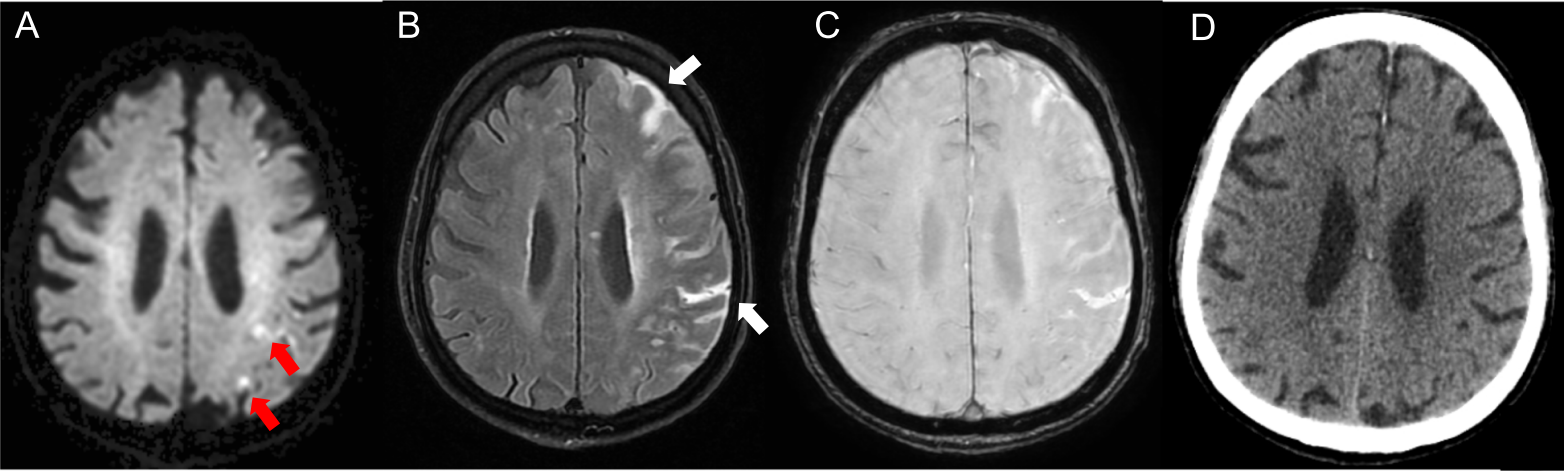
Day 2: Hyperintense Acute Reperfusion Marker Sign Associated with Acute Ischemic Lesions, Without Evidence of Subarachnoid Hemorrhage. A DWI sequence shows recent ischemic lesions (red arrows). B Non-enhanced FLAIR MRI sequence shows left hemispheric sub-arachnoid hyperintensity (white arrows). C SWAN MRI sequence shows no evidence of hemorrhage. D Brain CT shows no evidence of hemorrhage. DWI indicates diffusion-weighted imaging; FLAIR, fluid attenuated inversion recovery; MRI, magnetic resonance imaging; SWAN, susceptibility-weighted angiography
